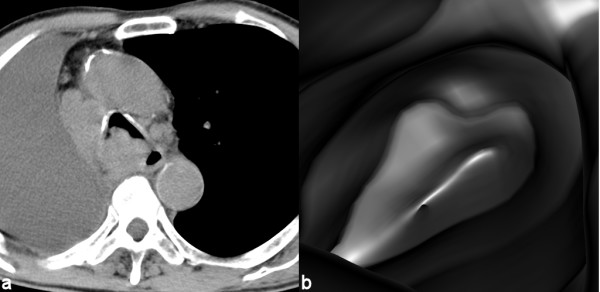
Tracheal invasion of adjacent lung cancer causing severe lumen stricture and distortion. Axial CT provides important information about the extraluminal structures; large fluid collection in the hemithorax, invasion and displacement of the tracheobronchial tree along with mediastinal lymphadenopathy; while reconstructed virtual endoscopic images demonstrate a distorted partially obstructed tracheal lumen. Distal navigation was only available with virtual endoscopy and provided also views of a compromised right main stem bronchus.
